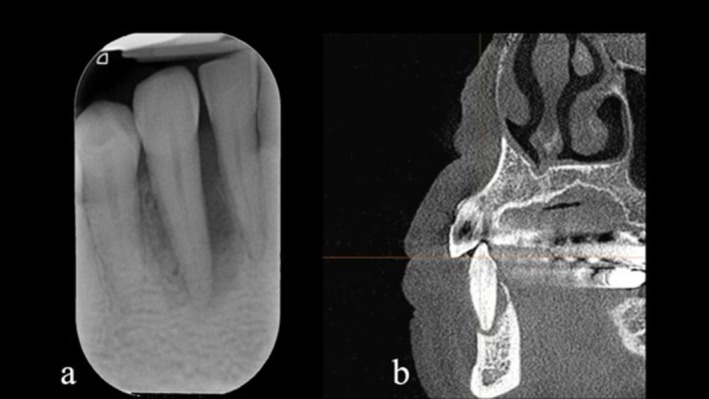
The periapical radiograph (a) and the corresponding sagittal CBCT section (b) display complete loss of the buccal bone plate and vertical bone loss exceeding beyond the root apex.
